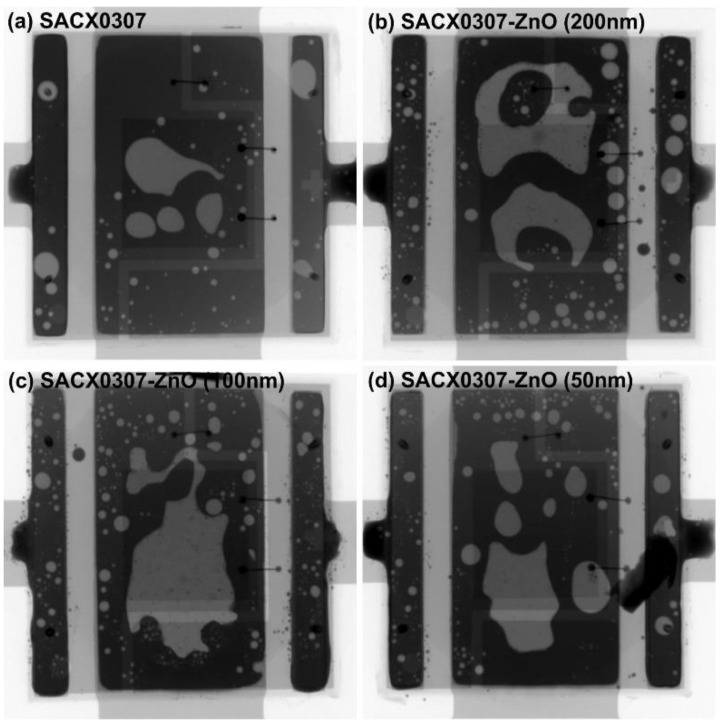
X-ray images of the different solder joints.
